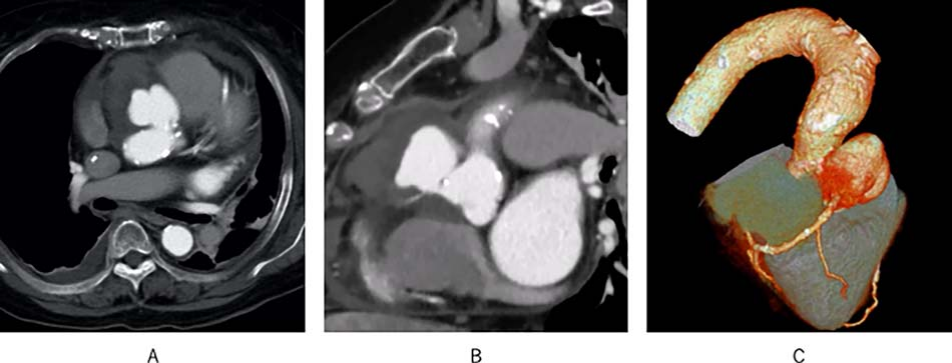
Preoperative contrast-enhanced CT; (A) a pseudoaneurysm was found in the aortic root; (B) the RCA appeared to originate from the pseudoaneurysm; (C) 3D CT showed the same results.
